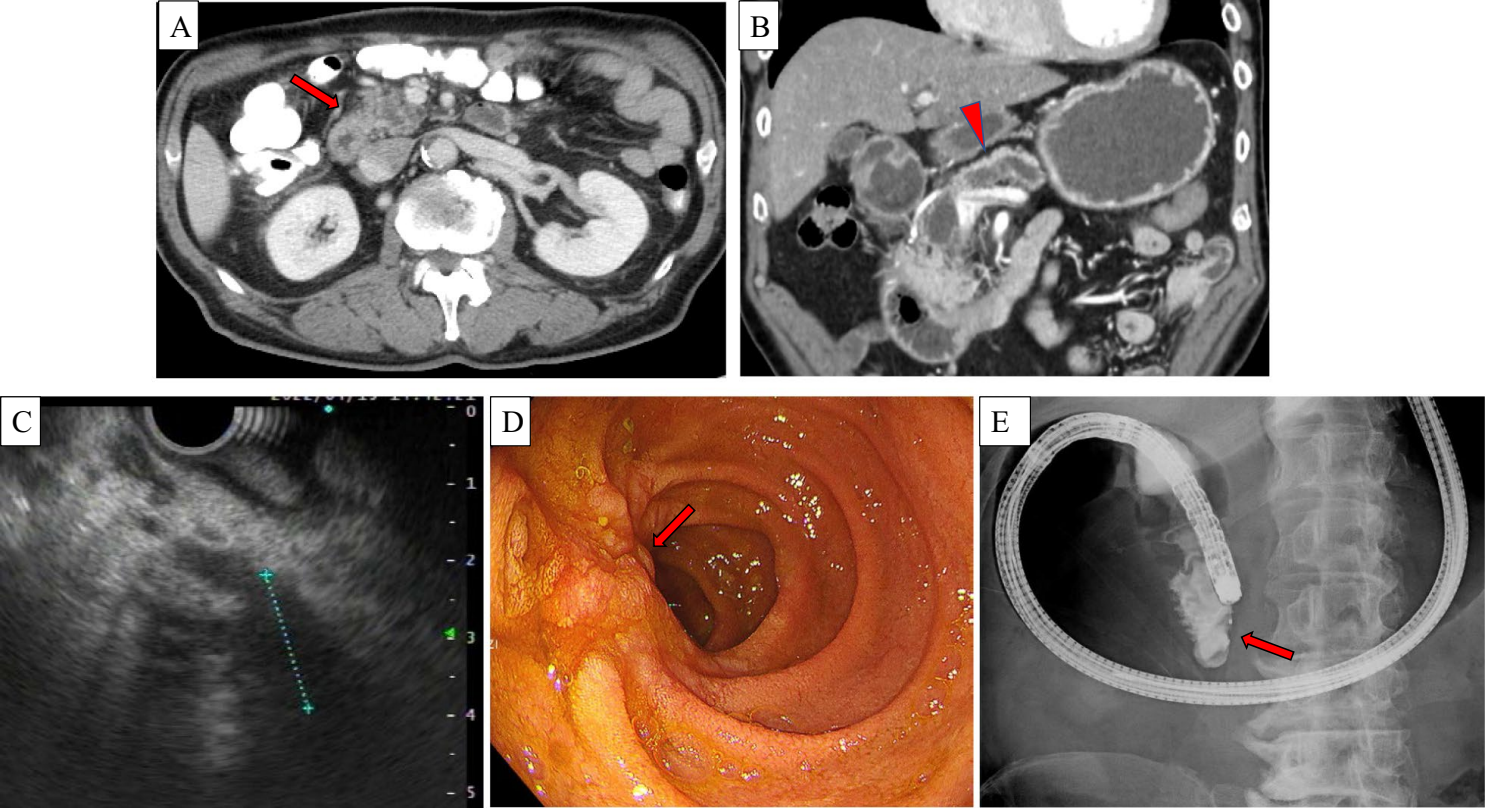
Enhanced abdominal CT and GI endoscopic findings. Enhanced CT showed stenosis in the third portion of the duodenum in the (A) (red arrow) axial view and (B) (red arrow) coronal view. Enhanced CT also revealed dilatation of the main pancreatic duct (B) (red arrowhead) and atrophy of the pancreatic head. EUS revealed a hypoechoic area at the deep lesion of pancreatic head, approximately 20 mm (C). GI endoscopy revealed one-third circumferential mucosal irregularity from the anal side of the main duodenal papilla to the inferior duodenal angulus, which bled easily (D) (red arrow). A biopsy was performed from the same site. Severe stenosis was observed from the inferior duodenal angulus to the third portion, and the scope could not pass through (E) (red arrow)
